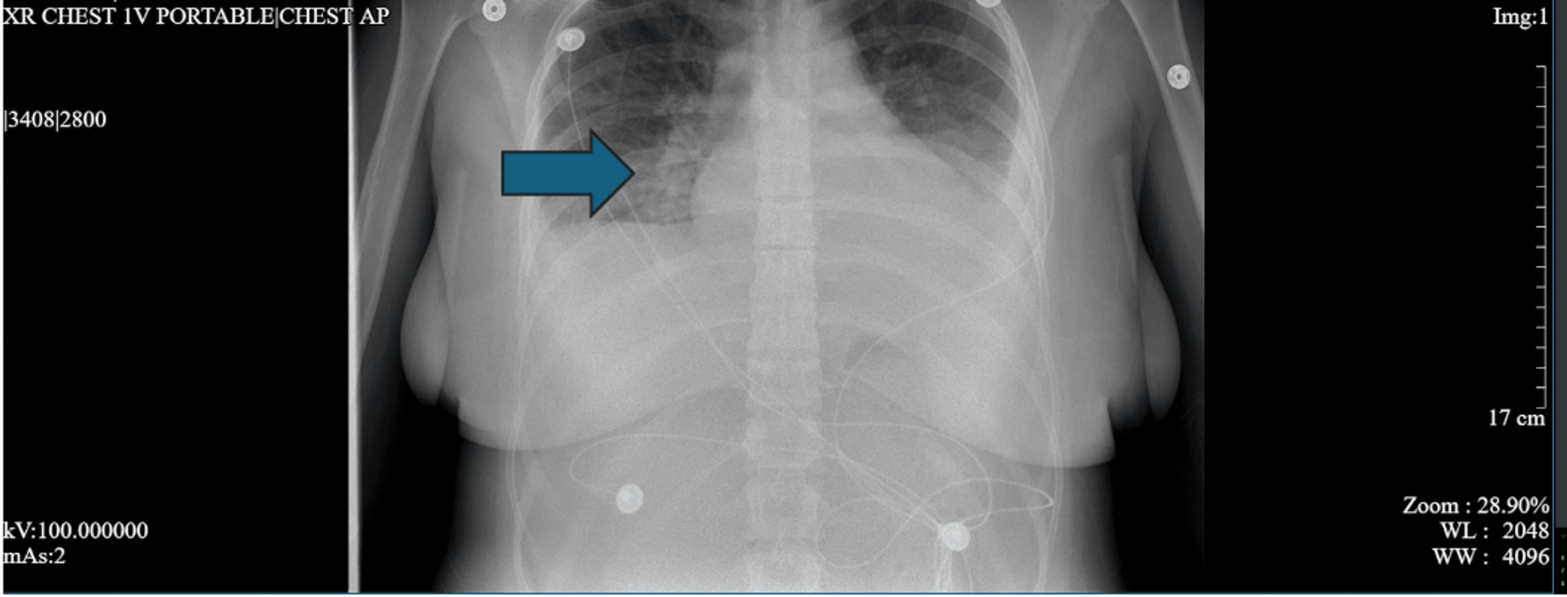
Chest X-ray taken on admission Arrow indicates the finding suggestive of right middle lobe consolidation
